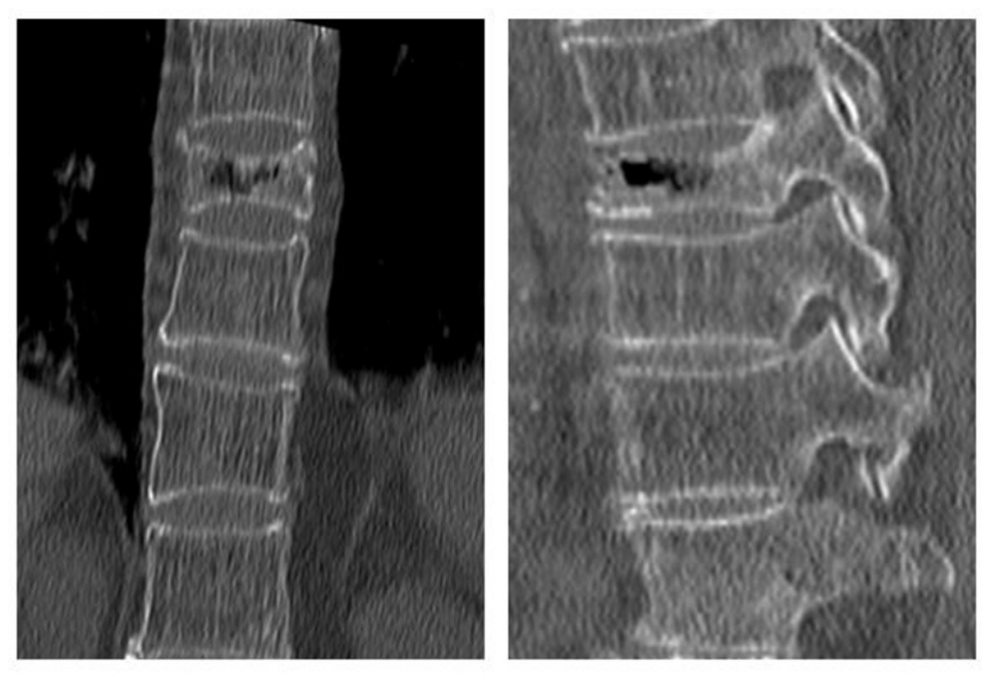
Intravertebral vacuum cleft (IVC) was demonstrated by computed tomography at L1 level.
